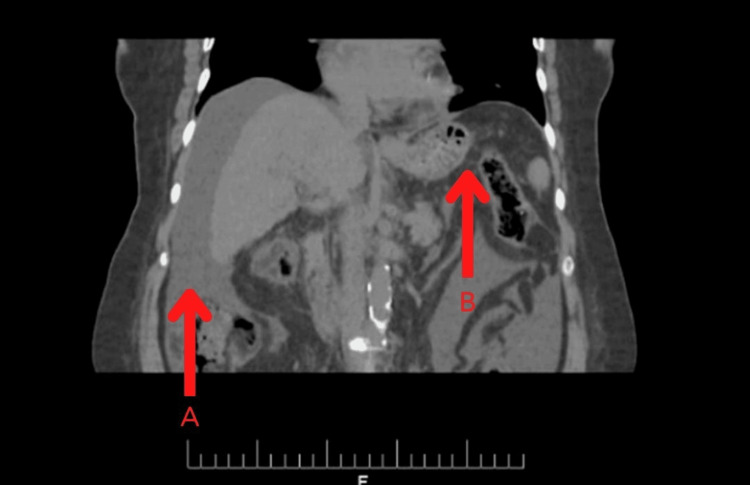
CT of the abdomen Moderate abdominopelvic ascites (A) and fat stranding were seen in the mesentery (B).
